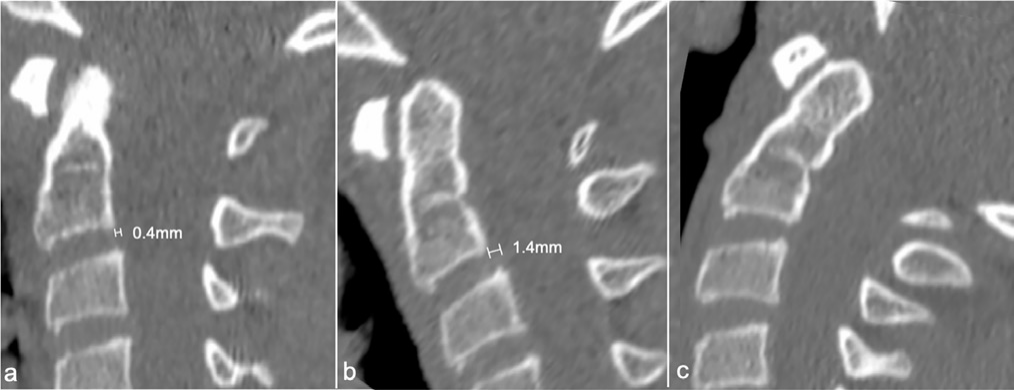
Sagittal CT reconstructed images of the upper cervical spine obtained from the 4D cine. (a)Neutral position, showing normal alignment of the craniocervical junction and minimal (0.4 mm, 2%) anterolisthesis of C2 on C3. (b)Flexion position showing normal alignment of the C1 posterior tubercle and slight increase in the C2/C3 anterolisthesis to 1.4 mm (7%). (c)Extended position showing no change in the alignment of the C1 posterior tubercle and resolution of the C2/3 anterolisthesis.
